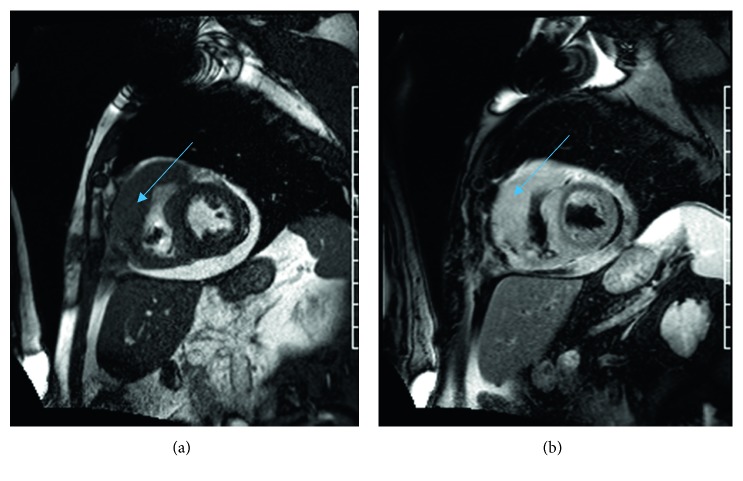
Cardiac MRI showing mass in lateral wall of the left ventricle on T1 and T2 imaging.
